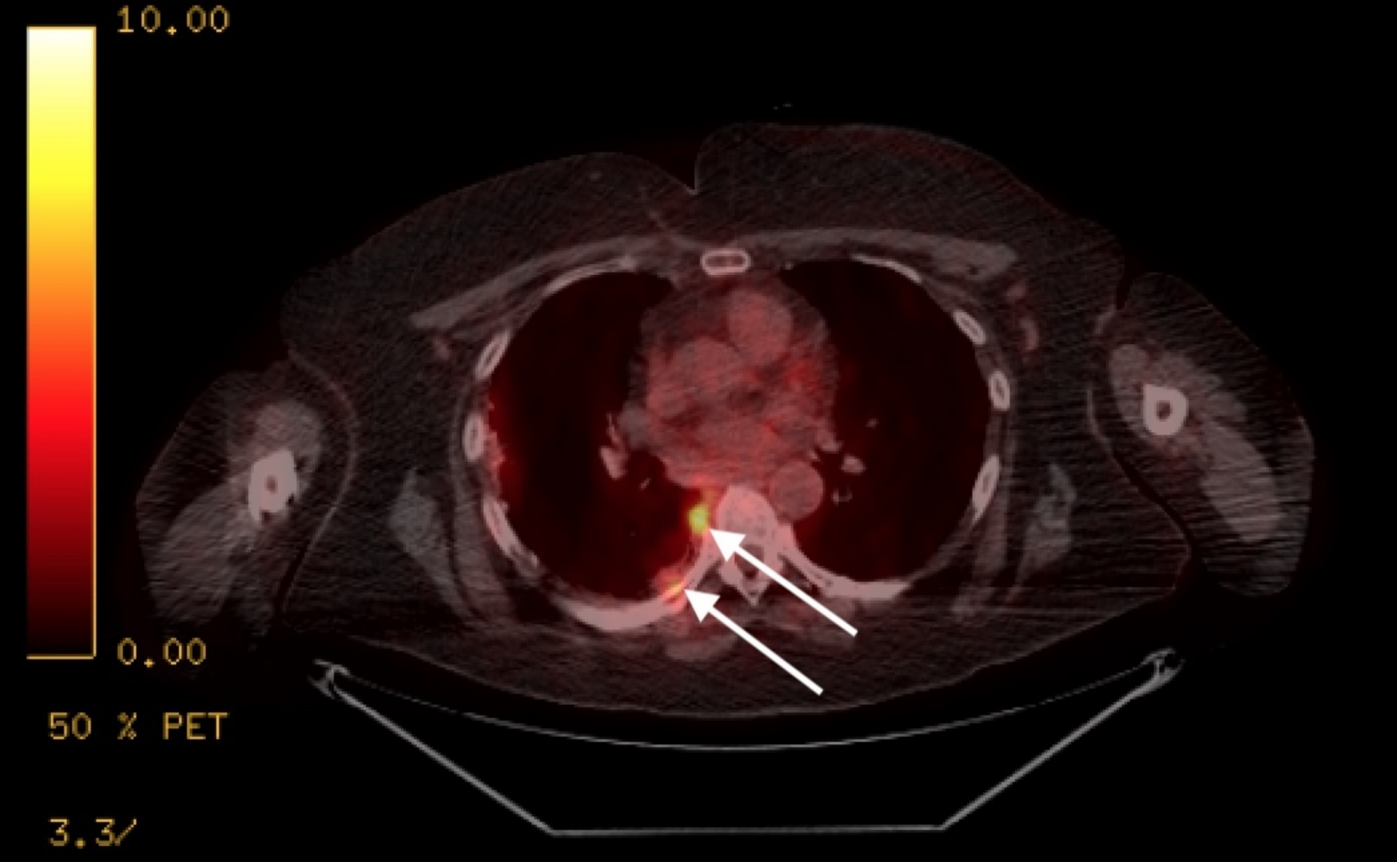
Positron emission tomography-computed tomography (PET/CT) image showing hypermetabolic pleural parenchymal activity along the periphery of the right lung.
